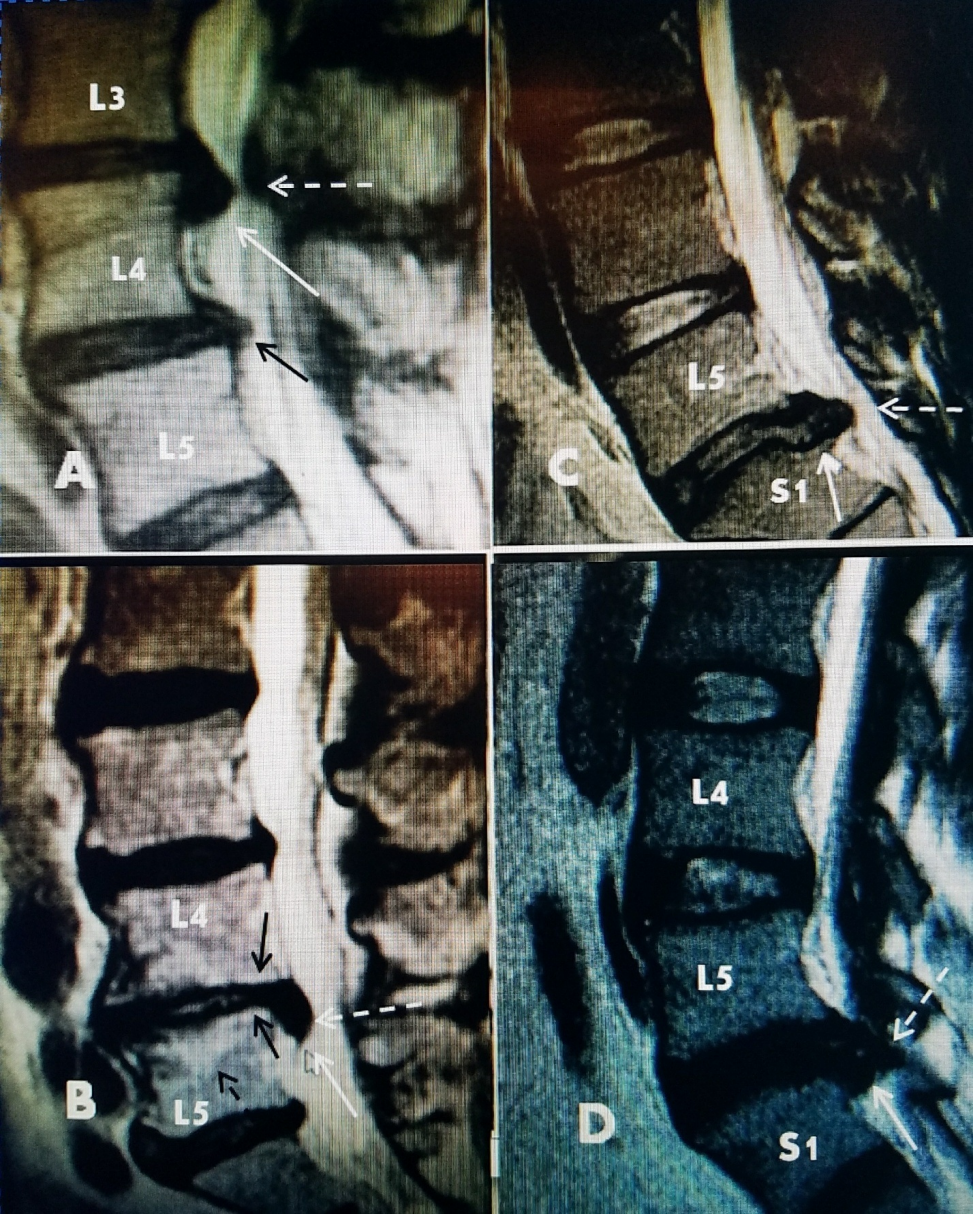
Examples of migrated and extruded disc fragments A: T2 sagittal magnetic resonance imaging (MRI) showing inferior extending L3-4 disc fragment (solid white arrow) and marked lateral spinal canal narrowing (dotted white arrow). There is also grade 1 spondylolisthesis at L4-5 with annular thickening (solid black arrow). B: Sagittal MRI showing inferior extending L4-5 disc fragment (solid white arrow) compressing and lifting the dura (dotted white arrow). There is associated L4-5 disc space narrowing (small solid black arrows) and early Modic endplate change especially at L5 (dotted short black arrow). C: T2 sagittal MRI showing large posteriorly extending L5-S1 disc (solid white arrow). The disc has marked T2 signal change and loss of fluid signal seen with disc dessication and fluid loss. The disc is extending directly posteriorly (dotted white arrow). D: MRI showing a large posterior lateral extrusion of L5-S1 disc (solid white arrow). The lateral recess is completely obstructed posteriorly adjacent to the facet joint (dotted white arrow).
